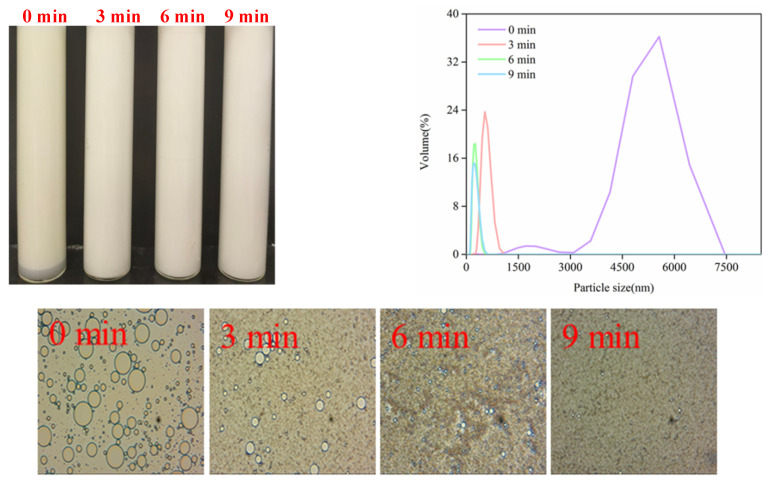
Effect of thermal treatment on the visible appearance, particle size distribution and microstructure of SC-coated O/W emulsions by a high-intensity ultrasound treatment.
